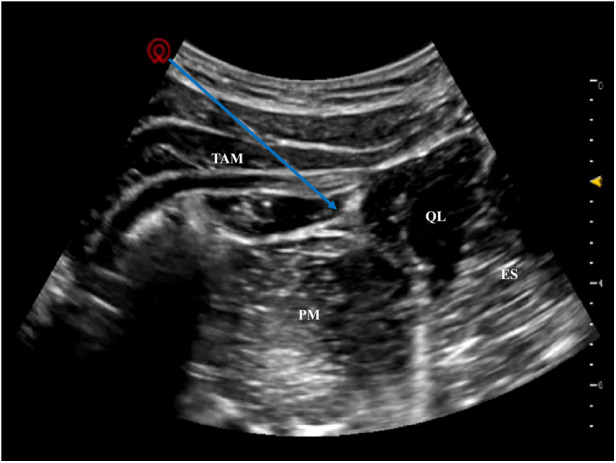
Ultrasound imaging of satisfactory 0.2% ropivacaine diffusion in the type Ⅱ QLB target space. Blue arrow indicates the diffusion range of the local anesthetic in the target fascial space, with no spread to adjacent blood vessels or abdominal organs.
